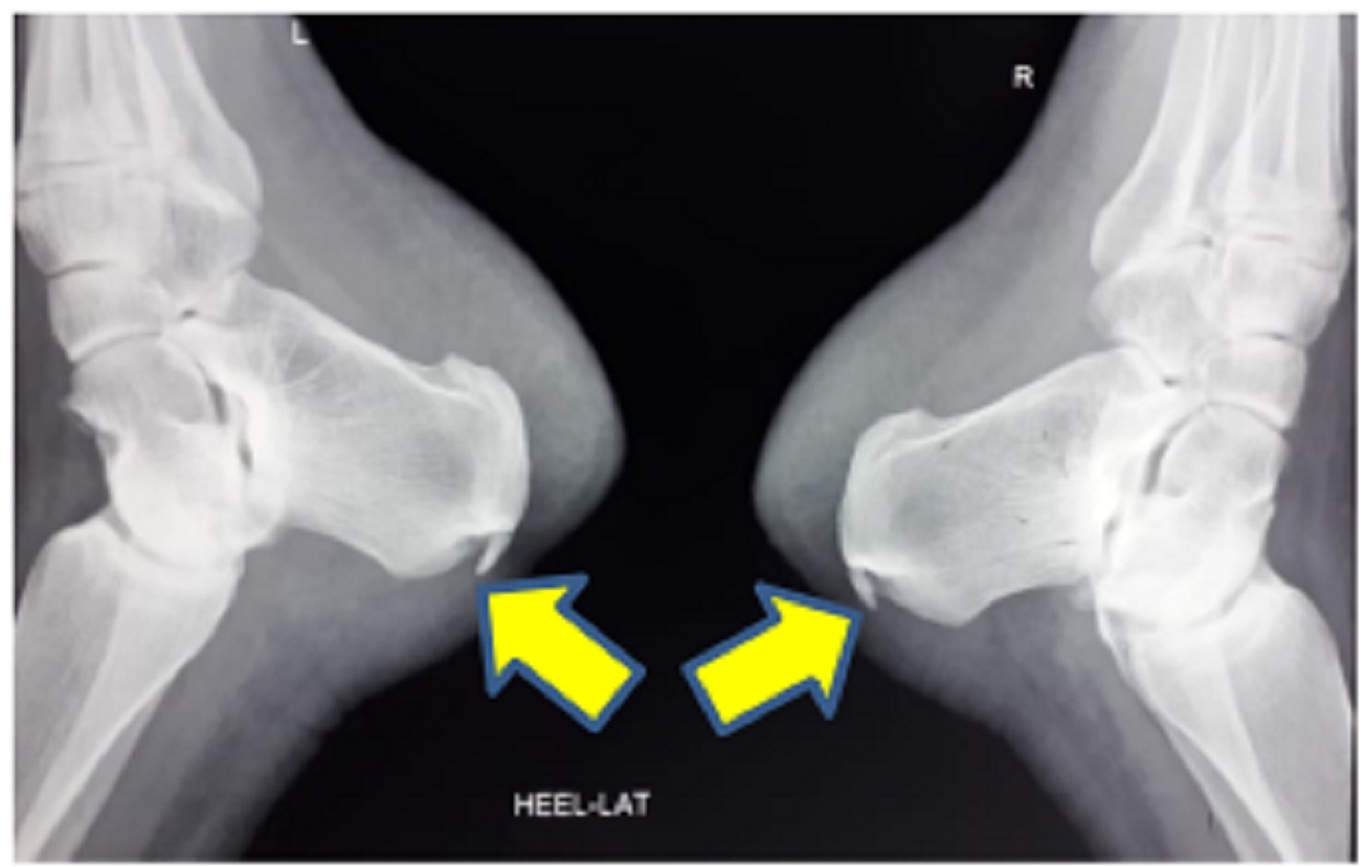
Lateral ankle radiograph showing bilateral Mulholland deformity
